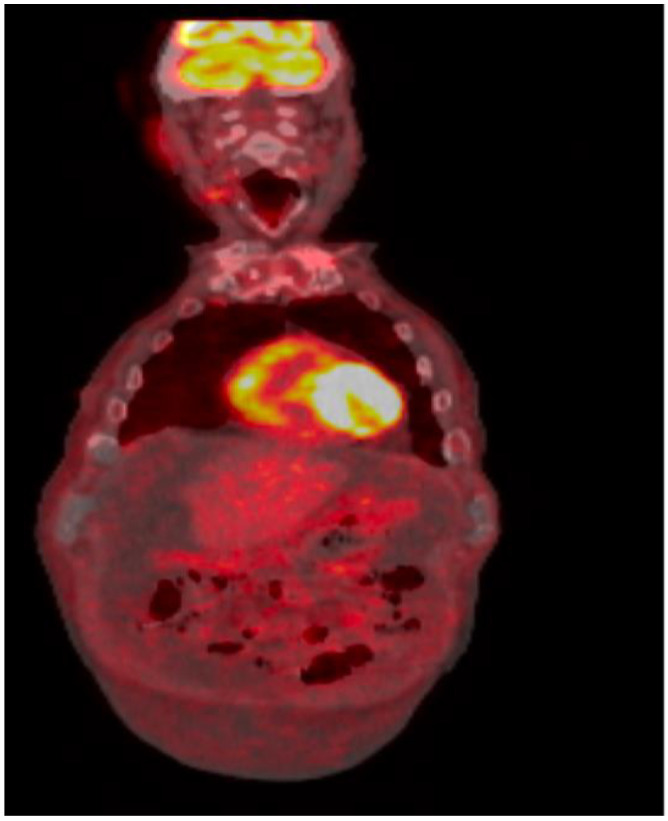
FDG-PET/CT: Large volume ascites with omental caking, the caking exhibited increased FDG activity with an SUV max of 3.1.
